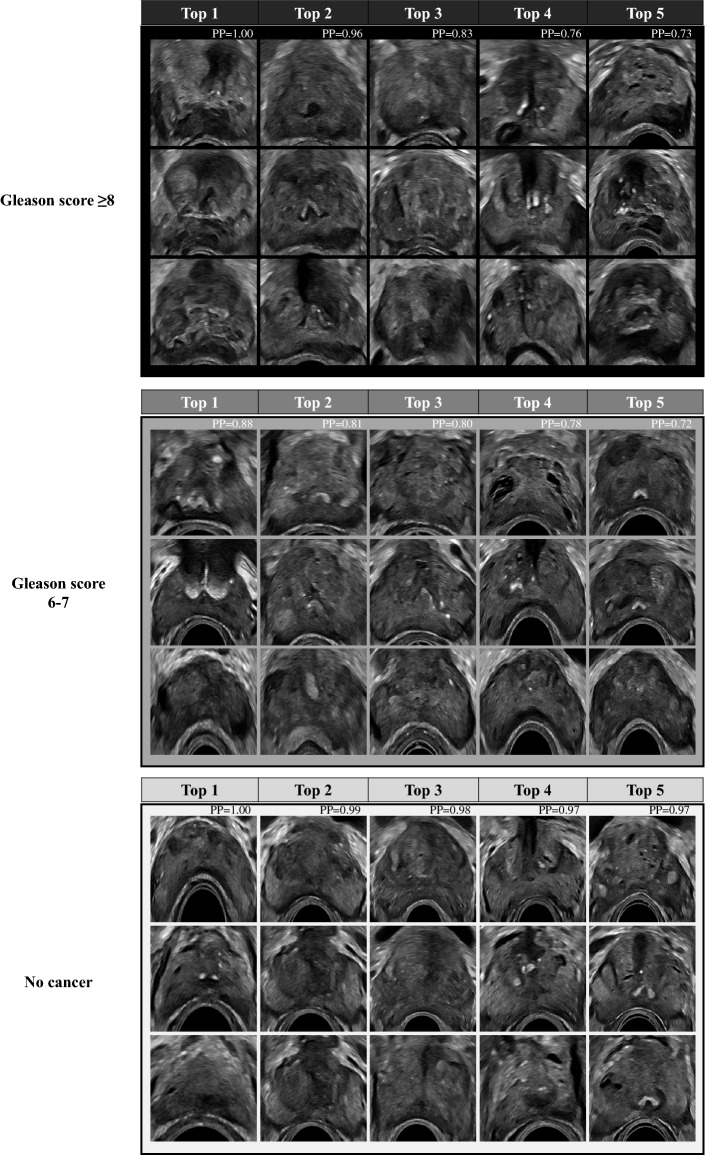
Prostate ultrasound images of top 5 cases corresponding to pathological cancer grading. Upper images: high-grade cancer cases. Middle images: low-grade cancer cases. Lower images: non-cancer cases. PP: normalized predicted probability.
